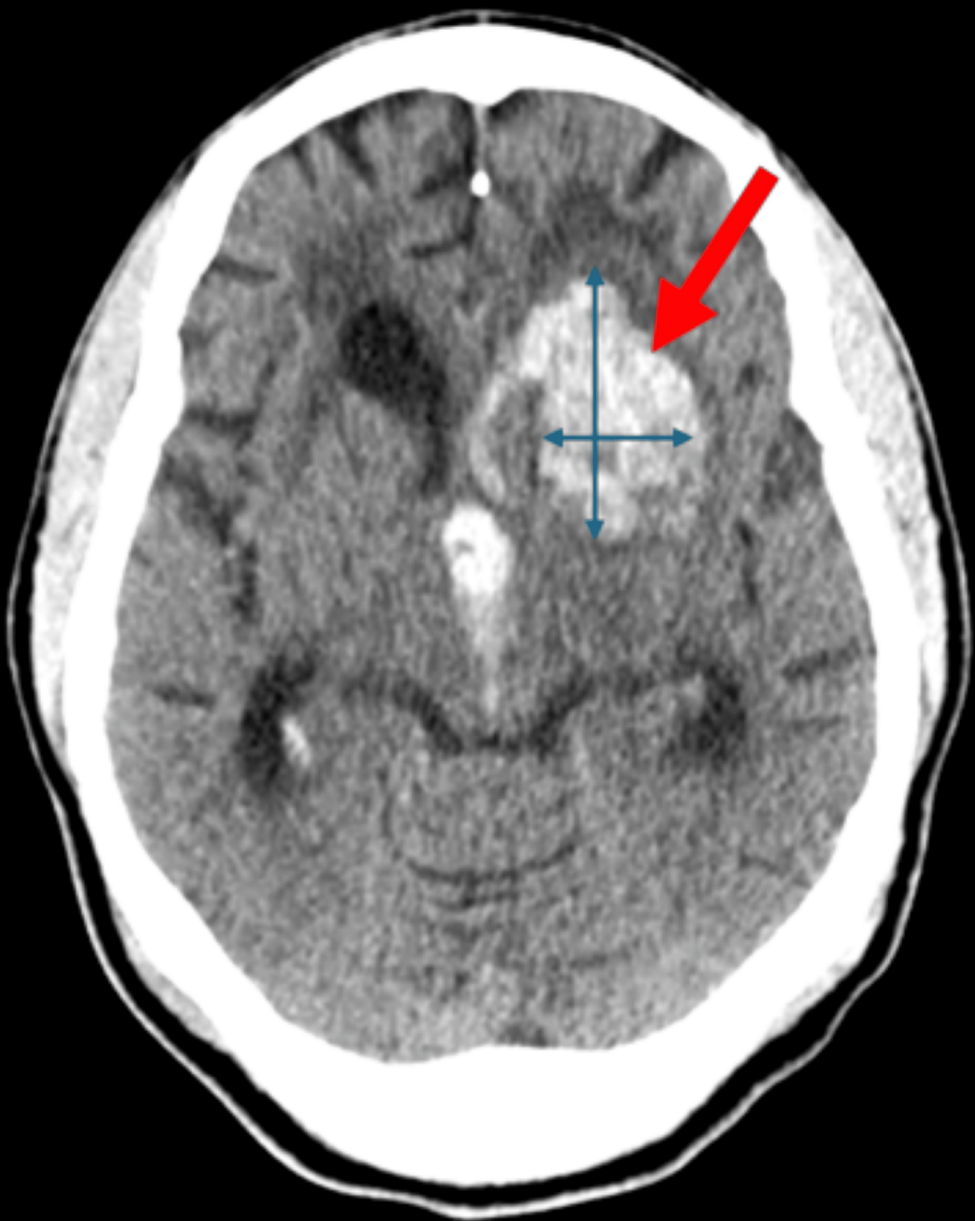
Axial non-contrast CT showing a left gangliocapsular intracerebral hemorrhage with surrounding edema and an associated rightward midline shift
